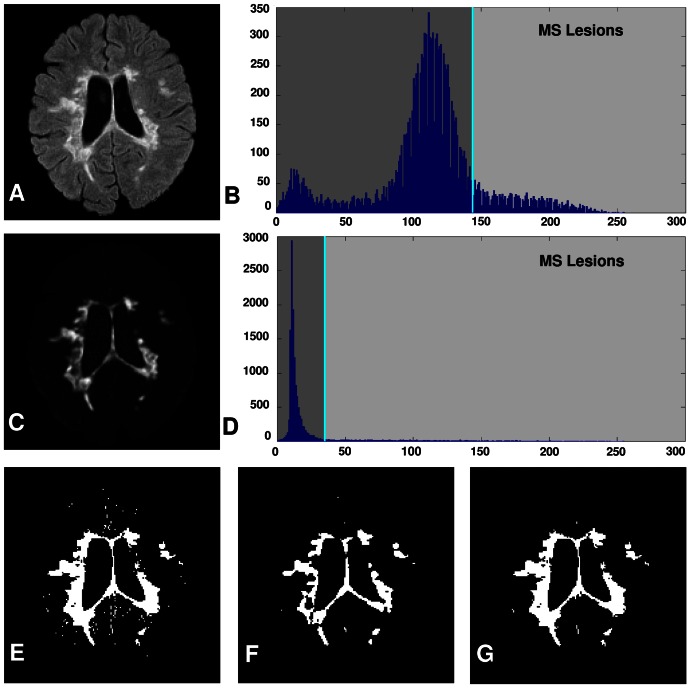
Contrast-Enhanced FLAIR image for segmentation of MS lesions. (a) Shows a typical brain image. (b) Histogram of original brain image. (c) Contrast-Enhanced image. (d) Histogram of Contrast-Enhanced image. (e)
